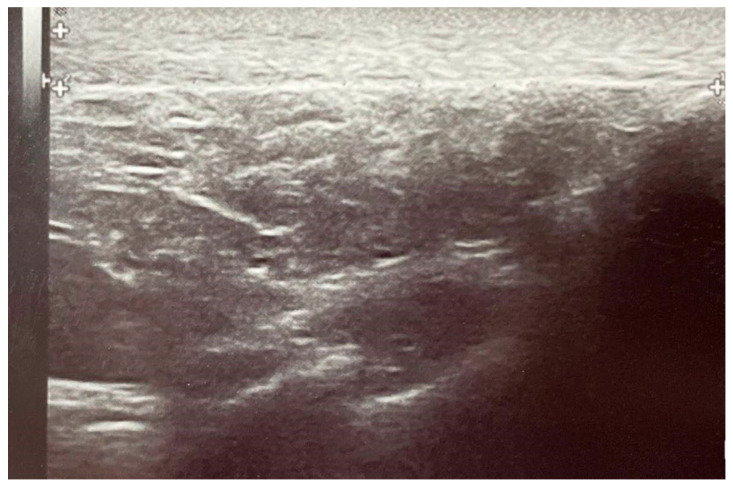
Longitudinal incidence ultrasound at treatment induction showing the physiological appearance of the Achilles tendon—diameter of 4.8 mm.
